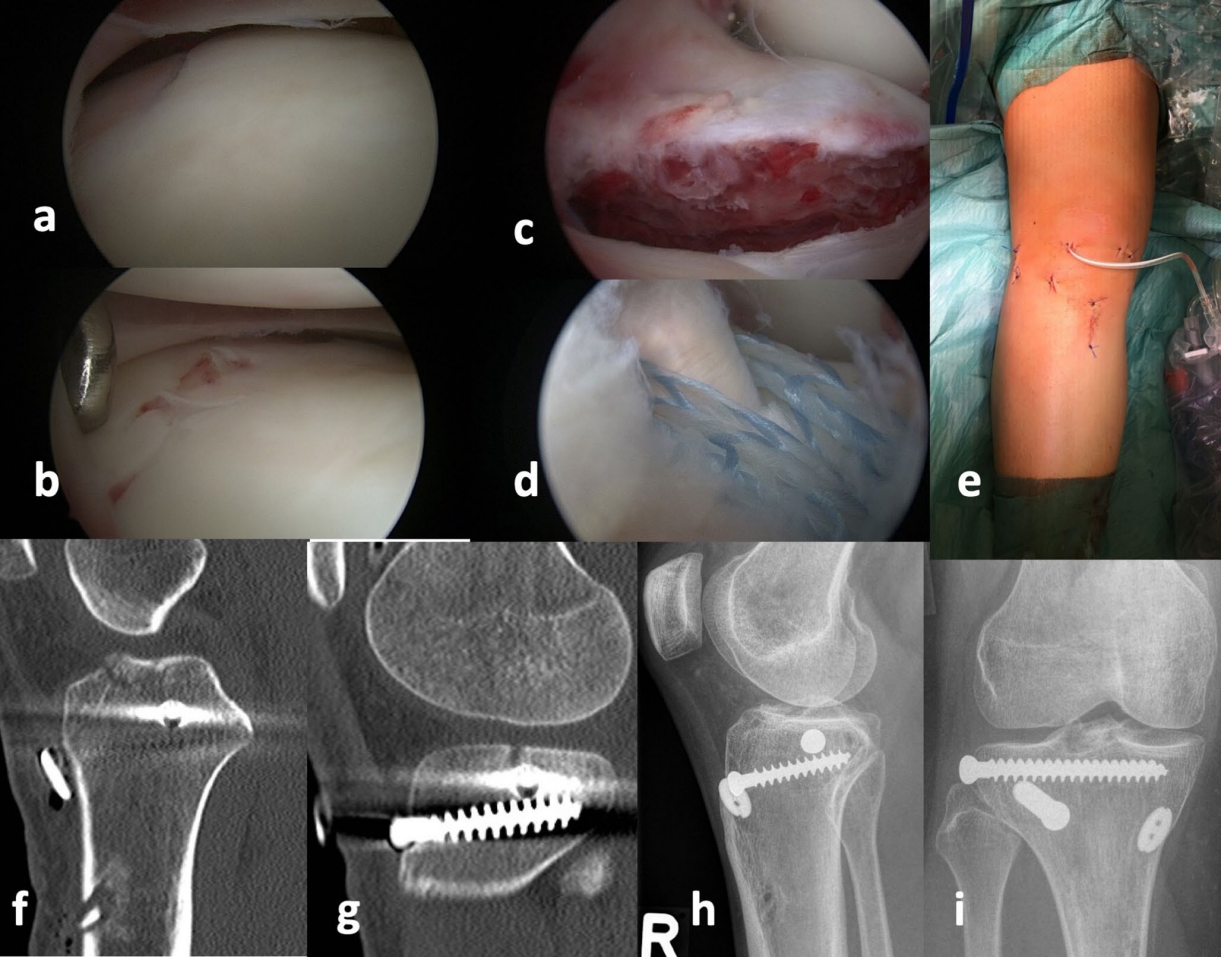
Posterolateral impression with accompanying dislocated eminence fracture. (a and b) demonstrate the initial impression and after reduction. (c) shows the dislocated bony avulsion of the ACL and (d) the fixation by using transosseous sutures. (e) demonstrates the less invasive treatment approach with minimal soft tissue compromise. Computed tomography was performed for postsurgical fracture position (f, g). X- rays at final follow up with complete fracture healing (h, i)
